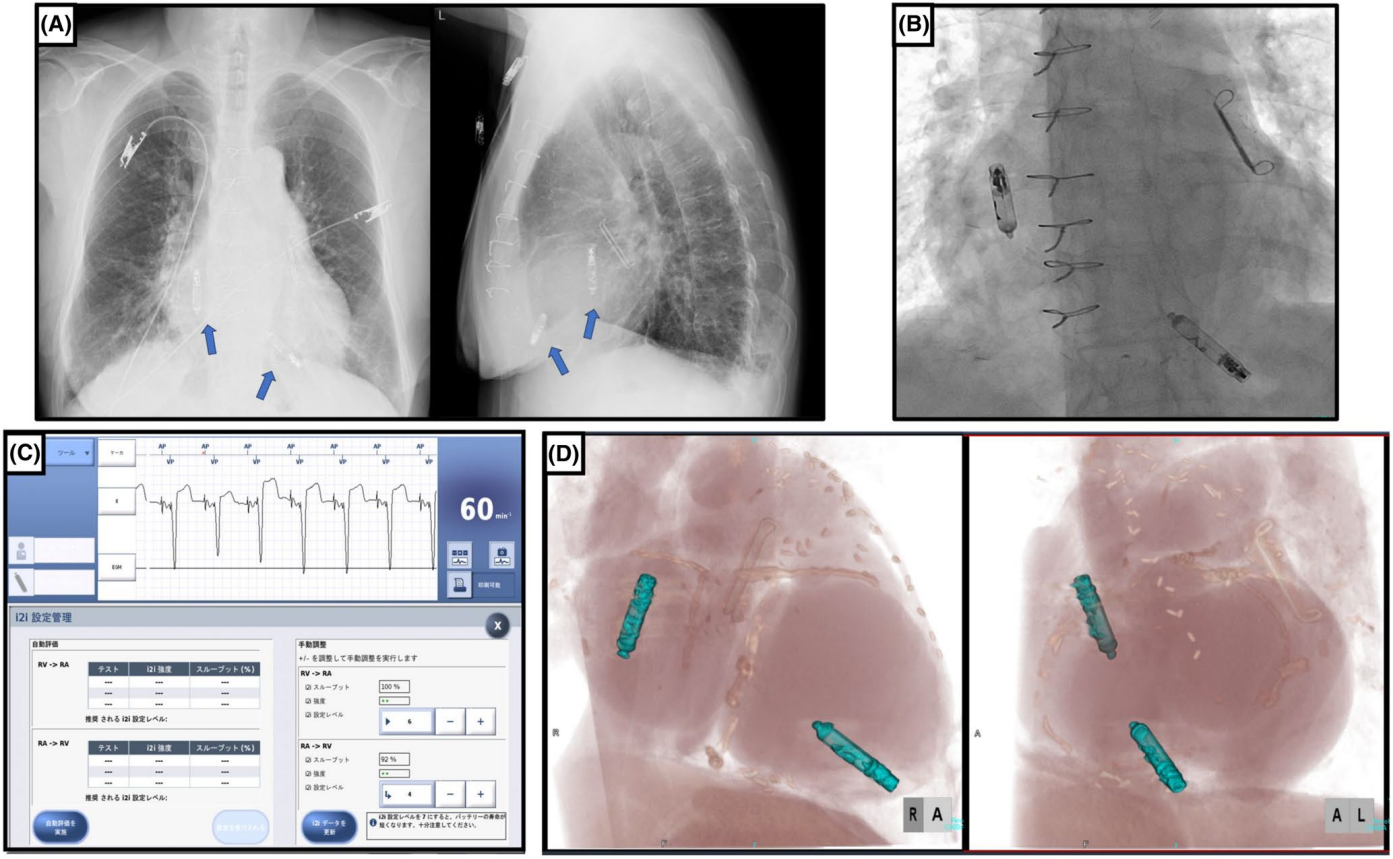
(A) Two‐view chest x‐ray of dual‐chamber leadless pacemaker. (B) Fluoroscopy image confirming successful implantation of Aveir AR and DR devices. (C) Intracardiac electrograms from the Aveir DR system showing appropriate sensing and pacing function. (D) Computed tomography image showing correct device positioning.
